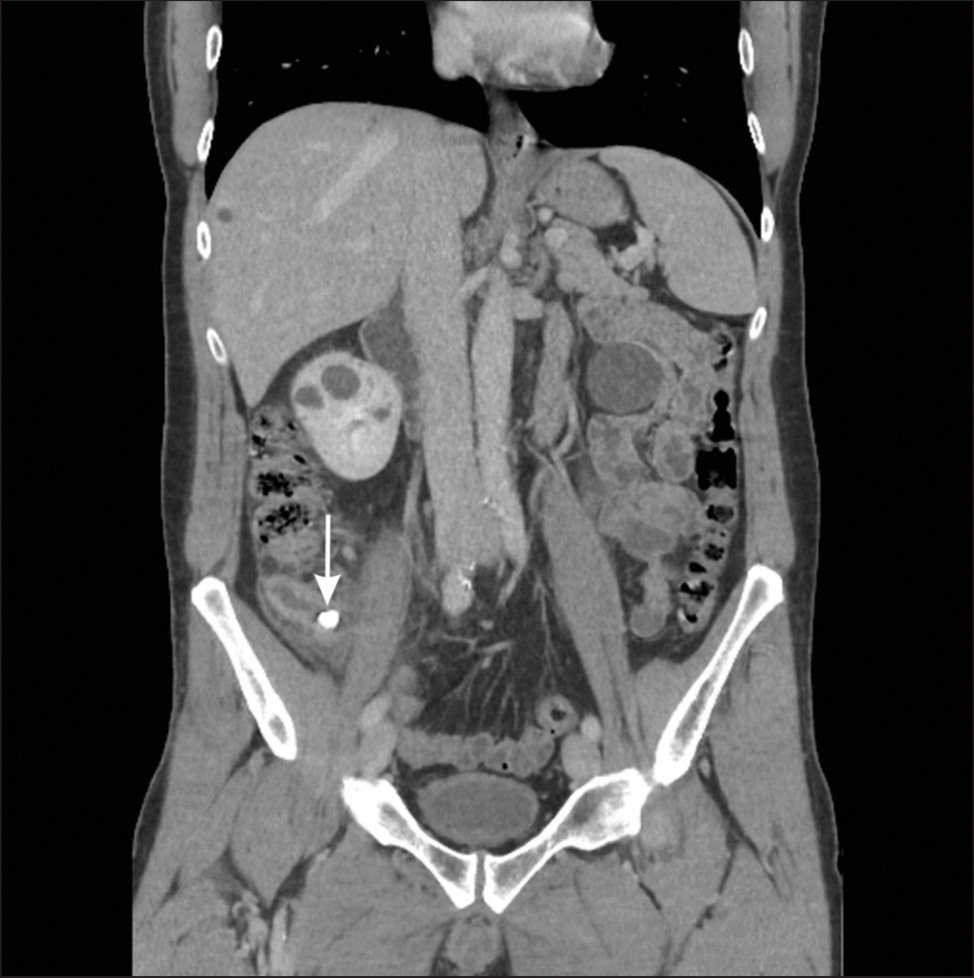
Coronal reconstruction of the initial contrast-enhanced CT performed at the acute onset of appendicitis. The appendix shows a thickened wall, fat stranding, free fluid and the embedded appendicolith. No obvious signs of a macroscopic interruption of the appendiceal wall was noted.
